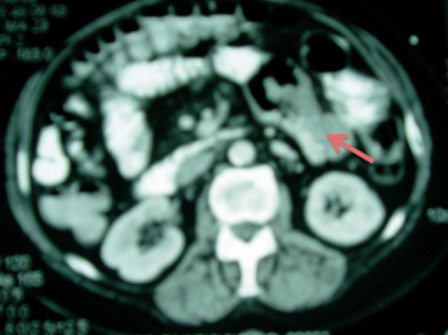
CT Abdomen showing the mass in the proximal small bowel.
